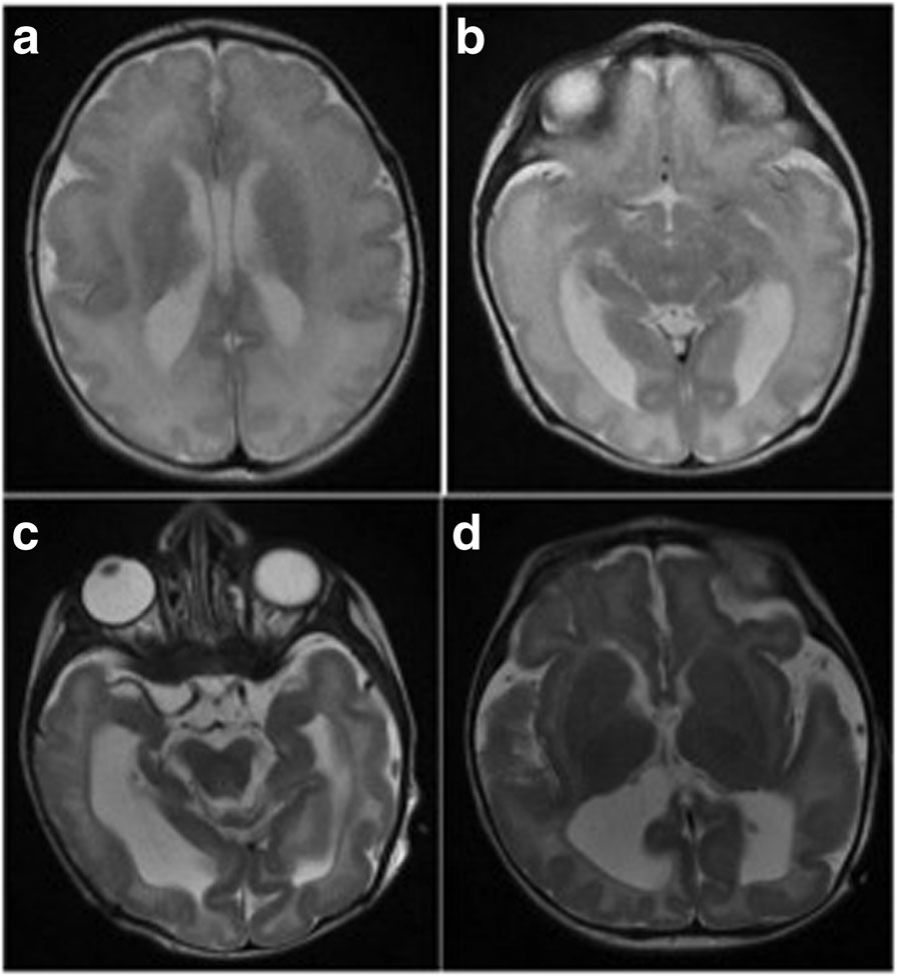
T2-weighted axial images of the cerebrum showing diffuse hyperintensity and atrophy of white matter (a, b, c, d), dilated ventricles (b, c, d), enlarged subarachnoid space (c, d), and partial agenesis of the corpus callosum (absence of splenium) (d)
